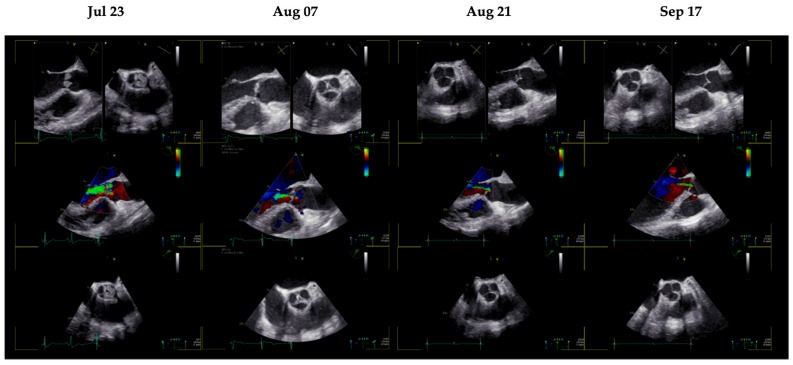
TEE examinations from initial diagnosis (23 July 2025) until complete resolution (17 September 2025). Significant regression is visible after 2 weeks of LMWH and targeted therapy (7 August 2025), with near-complete resolution by week 4 (21 August 2025), and confirmed complete resolution after 8 weeks (17 September 2025). TEE: transesophageal echocardiography. LMWH: low-molecular-weight heparin.
